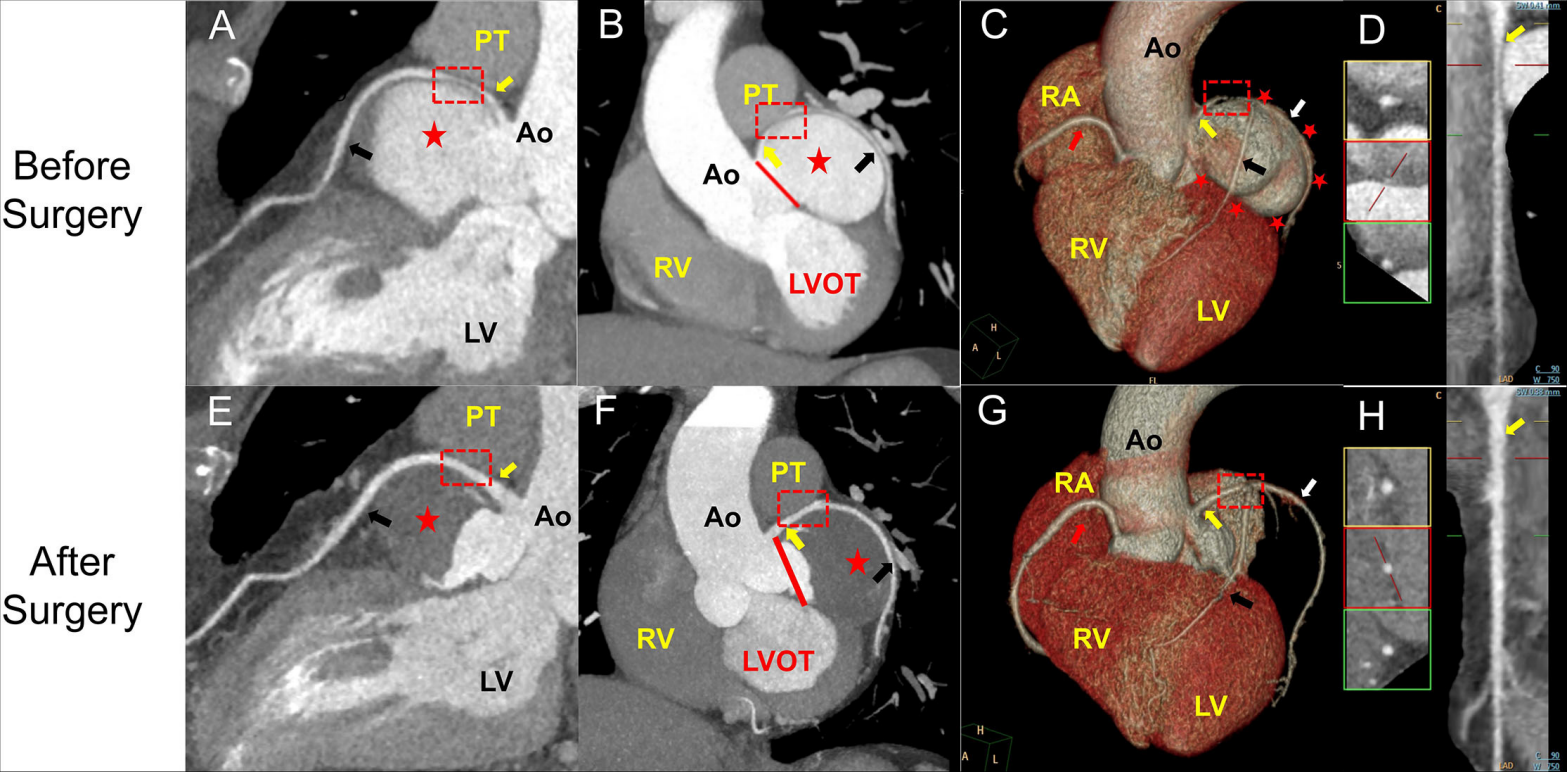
Coronary computed tomography angiogram (CCTA) images of the SoVA obtained before (A–D) and after (E–H) surgical treatment. (A,E) show a continuous curved vessel reconstruction of the left main and left anterior descending coronary arteries based on enhanced spiral CT before (A) and after the surgery (E). (B,E) show the CT coronal section of the reconstructed heart before (B) and after (F) the surgical exclusion. (C) shows a three-dimensional reconstruction model of the aneurysm before surgery, and (G) shows the same view absent the aneurysm after the surgical exclusion. (D,H) show the long-axis curvilinear reformation of the left main coronary artery (LMCA) before (D) and after the surgery (H). LMCA is identified with a red rectangle demonstrating extrinsic compression before surgery that has resolved after. The PT and LA were eliminated in (C,F) to show the LMCA. LMCA, left main coronary artery (yellow arrow); LAD, left anterior descending coronary artery (black arrow); PT, pulmonary trunk; LV, left ventricle; Ao, aorta; RV, right ventricle; LVOT, left ventricle outflow tract; LV, left ventricle; red star, SoVA; red line, the neck of the aneurysm; red arrow, right coronary artery; white arrow, left circumflex coronary artery.
